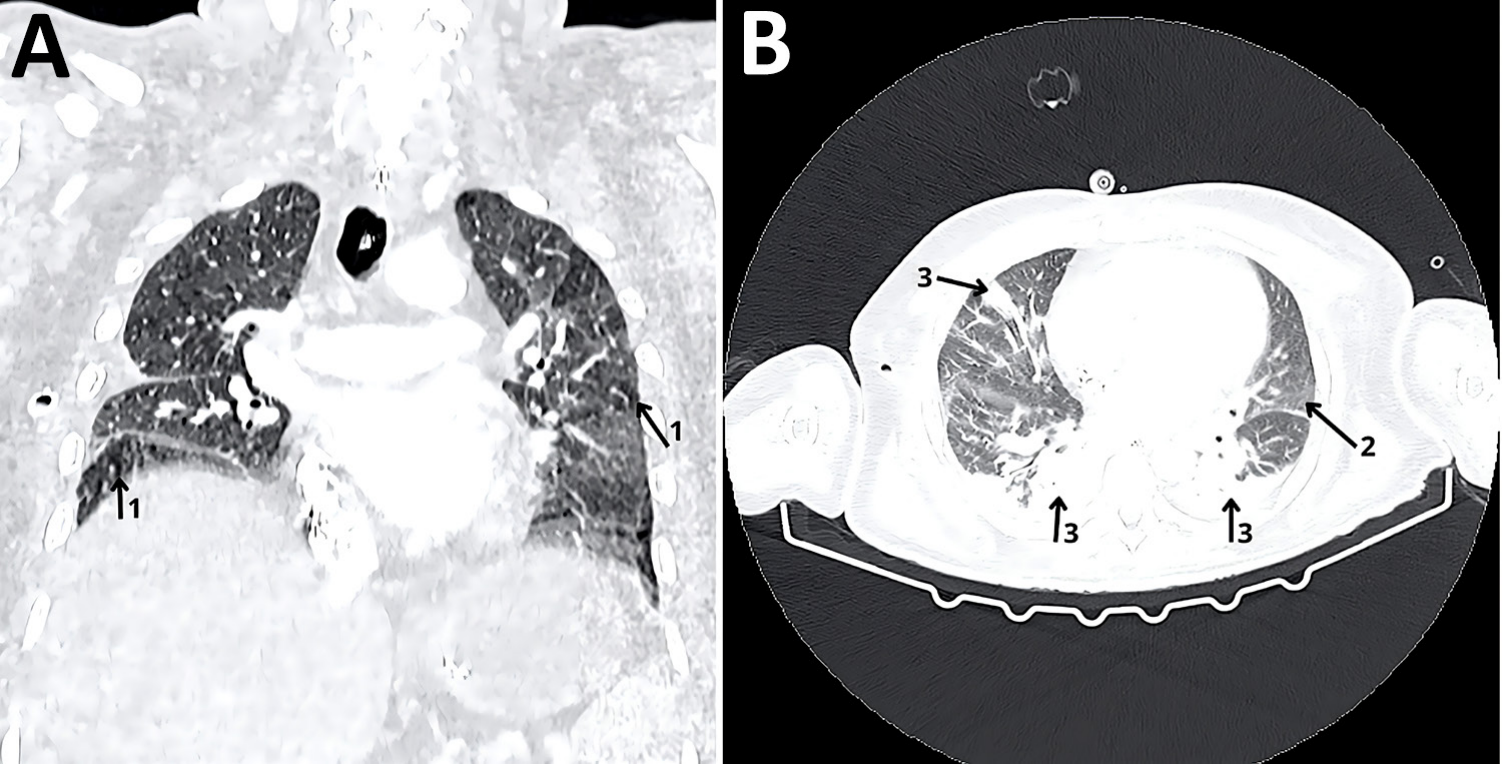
Computed tomography with contrast of the chest of a patient from Brazil infected with *Burkholderia mallei*. A) Coronal section, showing a heterogeneous pattern of lung attenuation. Arrows marked 1 indicate a suggestive disturbance in the ventilation-perfusion relationship. B) Axial section. Arrow marked 2 indicates consolidative opacities. Arrows marked 3 indicate subsegmental atelectasis in both lungs.
